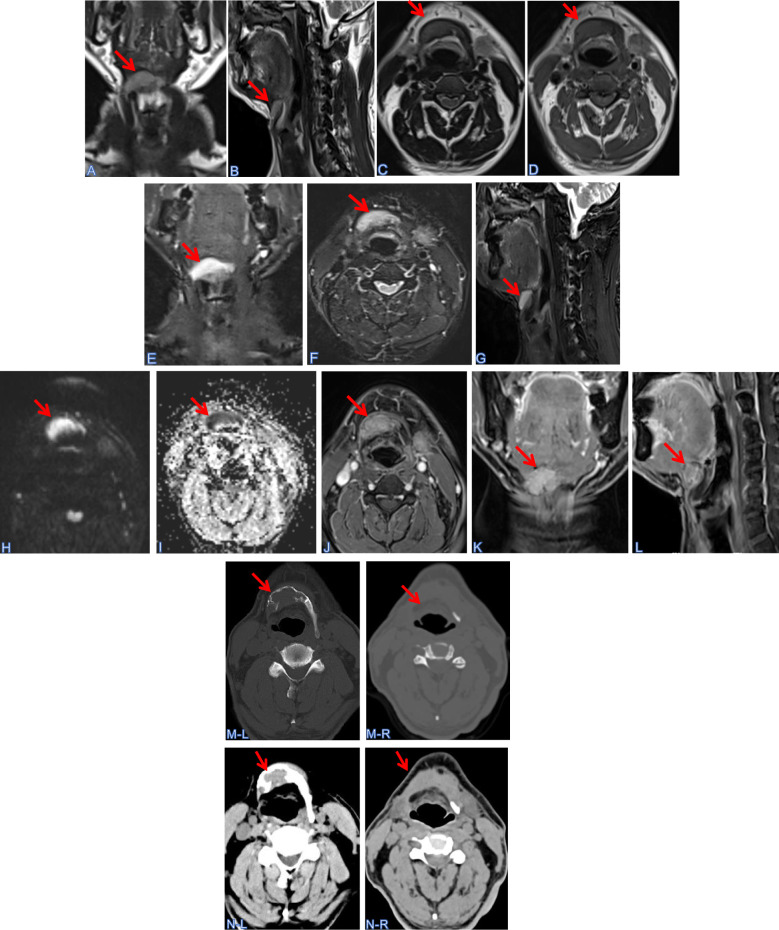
MR&CT imaging of head and neck masses. (A-D) Contrast-enhanced MR imaging: Expansive bone destruction of the hyoid bone to the right. (A) Coronal T2W1; (B) transverse T1W1; (C) sagittal T2W1; (D) transverse T2W1; (E-G) FS; (H) DWI; (I) ADC; (J-L) Multilocular expansive bone destruction of the hyoid bone. The tumor is approximately 3.3 cm×1.5 cm in size, with punctate calcification and a bone ridge. The bone cortex was discontinuous at the edge of the lesion, and soft tissue protrusion was observed. The lesions showed mild enhancement on contrast-enhanced scans. (M) Bone window in transverse axis view. (N) Soft window the transverse in axis position. (Left: before surgery; Right: 2 months after surgery).
